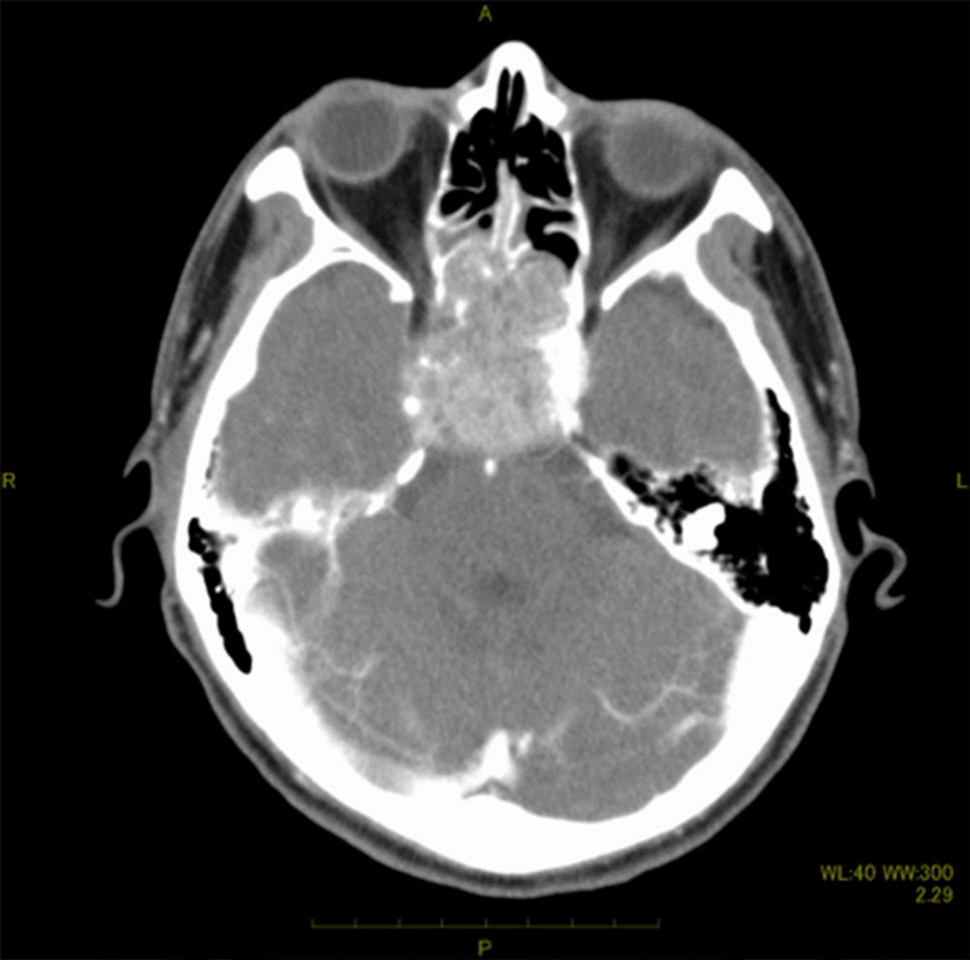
Contrast-enhanced computed tomography scan images revealed a tumor that had spread bilaterally from the sphenoid sinus to the ethmoid sinus and showed multiple honeycomb-like low-density areas and suggested skull base infiltration
